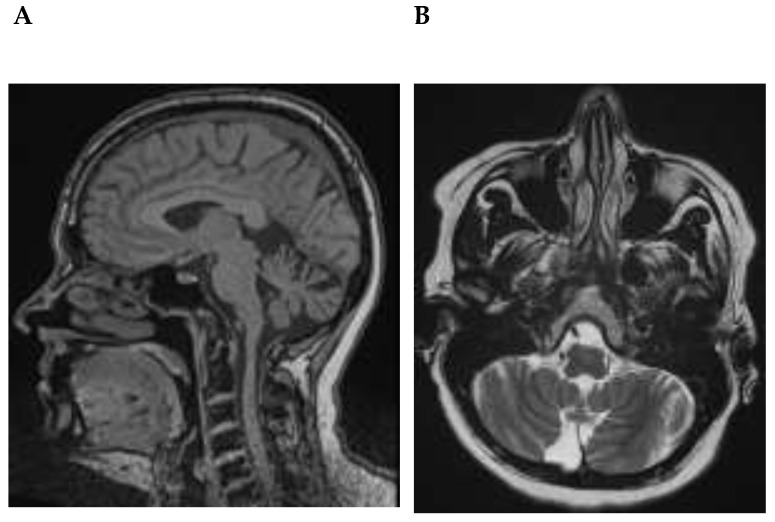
Cerebellar atrophy in a patient associated with Homer-3 autoantibodies. (A) Sagittal T1-weighted magnetic resonance imaging (MRI) and (B) transversal T2-weighted MRI showing cerebellar atrophy.
